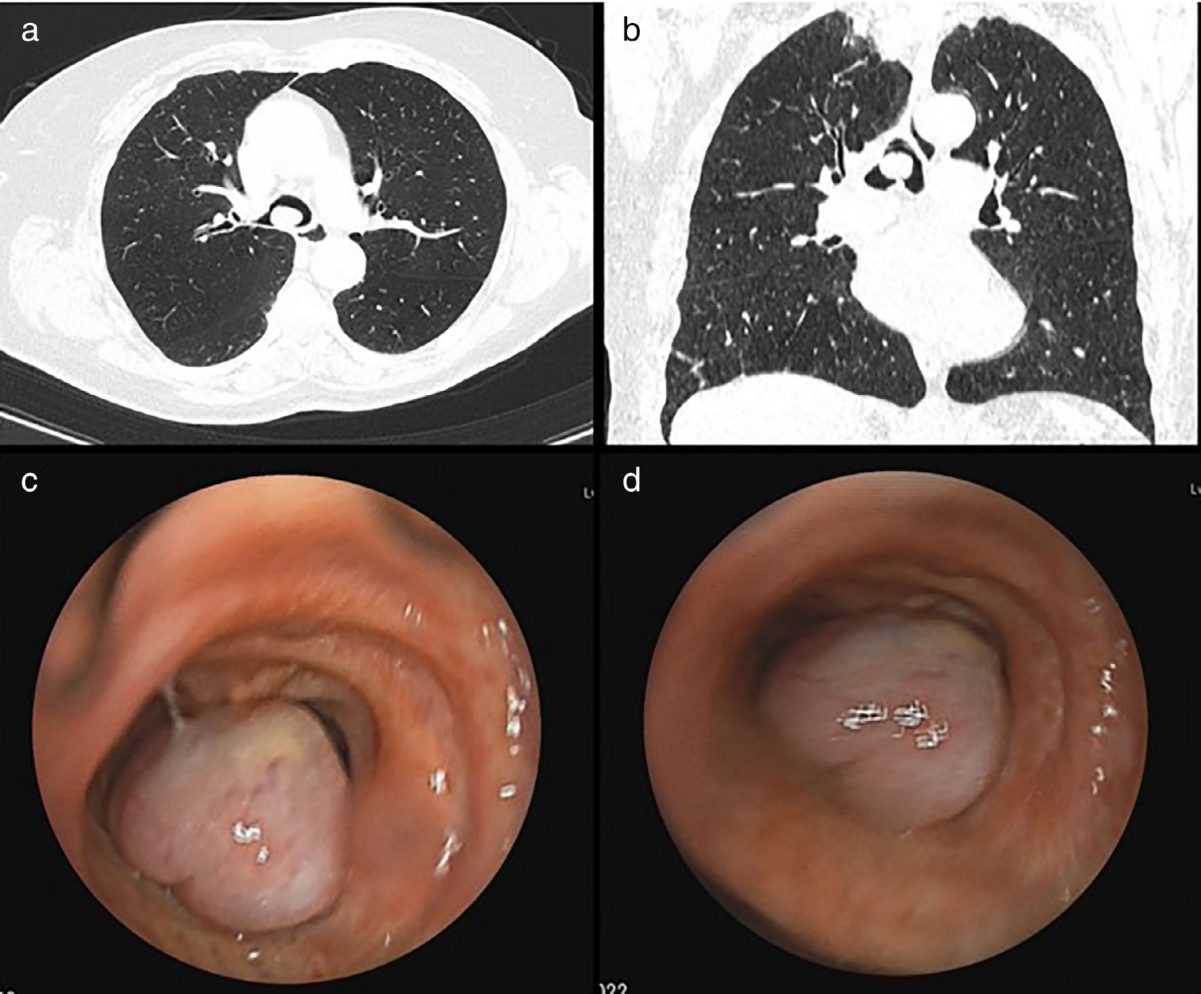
Axial and coronal contrast computed tomography (CT) (a, b) and endoscopic view during rigid broncoscopy (c, d) showing the presence of a tracheal pedunculated polyp lesion with a thin implantation base on the carina
